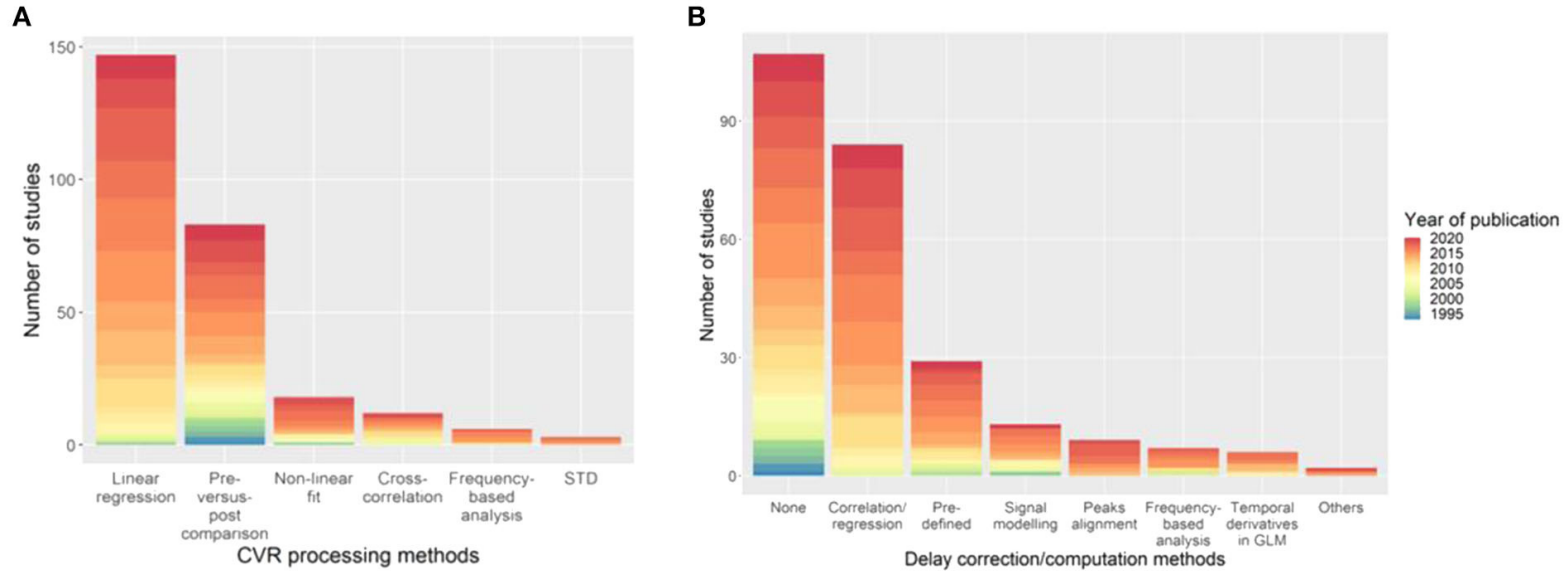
Distribution of the (A) CVR processing and (B) delay computation methods with the associated year of publication of the paper. The category “Others” in (B) includes deconvolution to find the HRF between the EtCO_2_ and the MRI signal, and GLM with two (“fast” and “slow”) regressors. STD, standard deviation of MRI signal; HRF, haemodynamic response function; GLM, general linear model.
